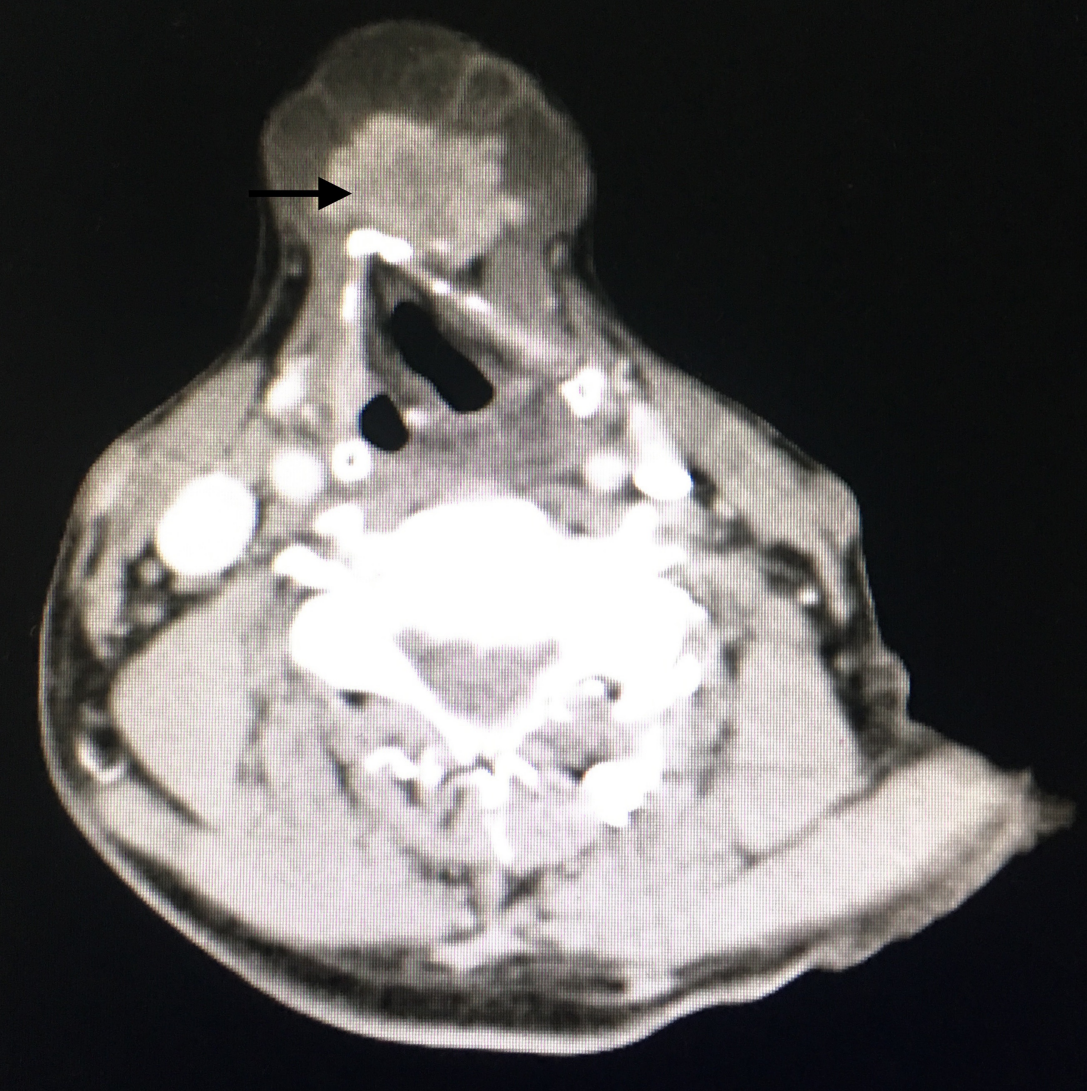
Contrast-enhanced CT scan showing a multiseptated cystic lesion with enhancing mural nodule anterior to the thyroid cartilage (arrow).
